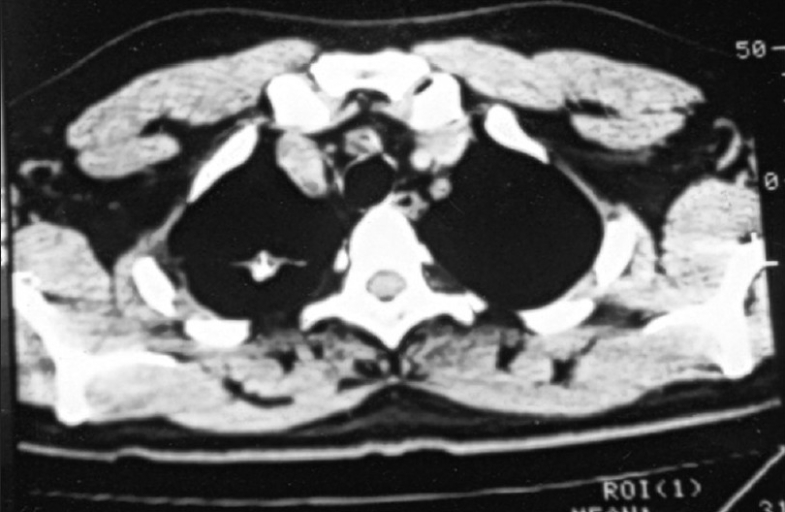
CT scan thorax showing a nodular lesion (tuberculosis) at the right upper lobe
